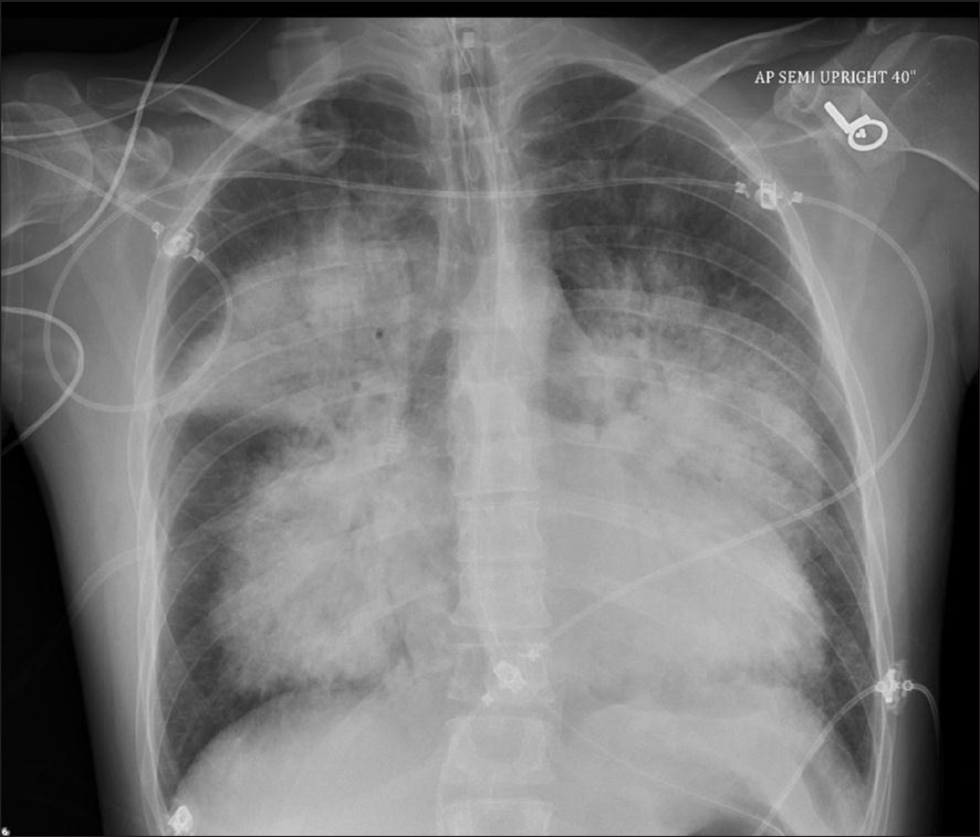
Chest x-ray upon presentation showing extensive dense perihilar mass-like consolidative opacities.
